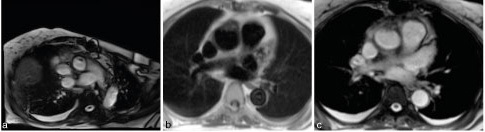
Cardiovascular MR. (a) Transaxial steady-state free precession cine of the ascending aorta shows the intraluminal mobile mass. (b) Axial slice of axial spin echo (half-Fourier acquisition single-shot turbo spin-echo) sequence at the same position as the CT image in Figure 3. The irregularly thickened anterior wall of the aortic graft with intraluminal components is noteworthy. (c) Axial slice of gradient echo (true fast imaging with steady-state free precession) sequence at the same position as the previous image confirms the irregularly thickened anterior wall of the aortic graft with vegetation extending into the lumen.
